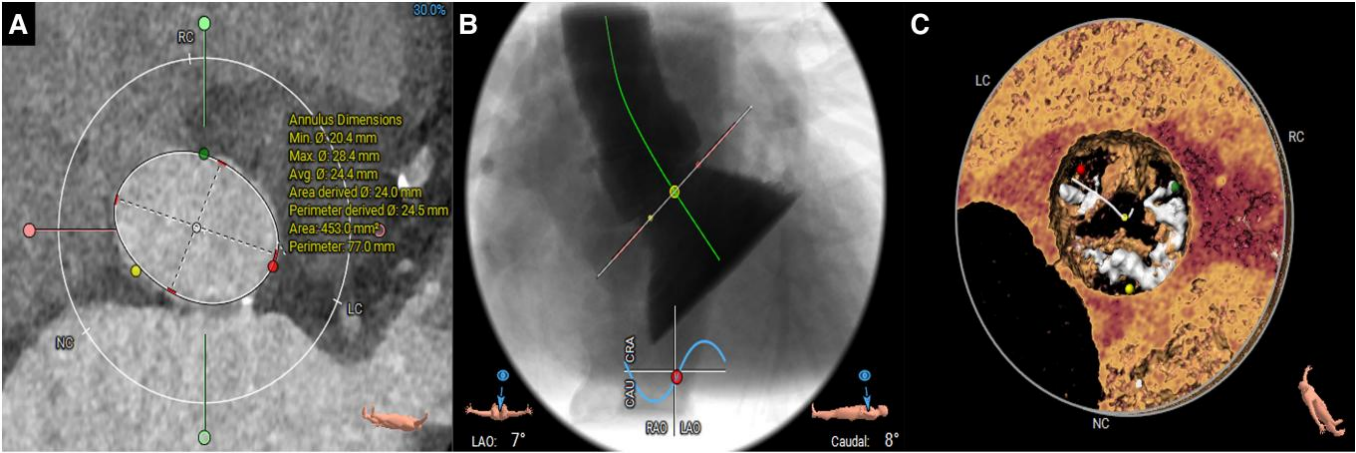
Multidetector computed tomography images of the native aortic valve. (*A*) Measurement of aortic annulus for cross-sectional area and perimeter in end systole. (*B*) Three-cusp coplanar view. (*C*) Three-dimensional reconstructions of valve.
